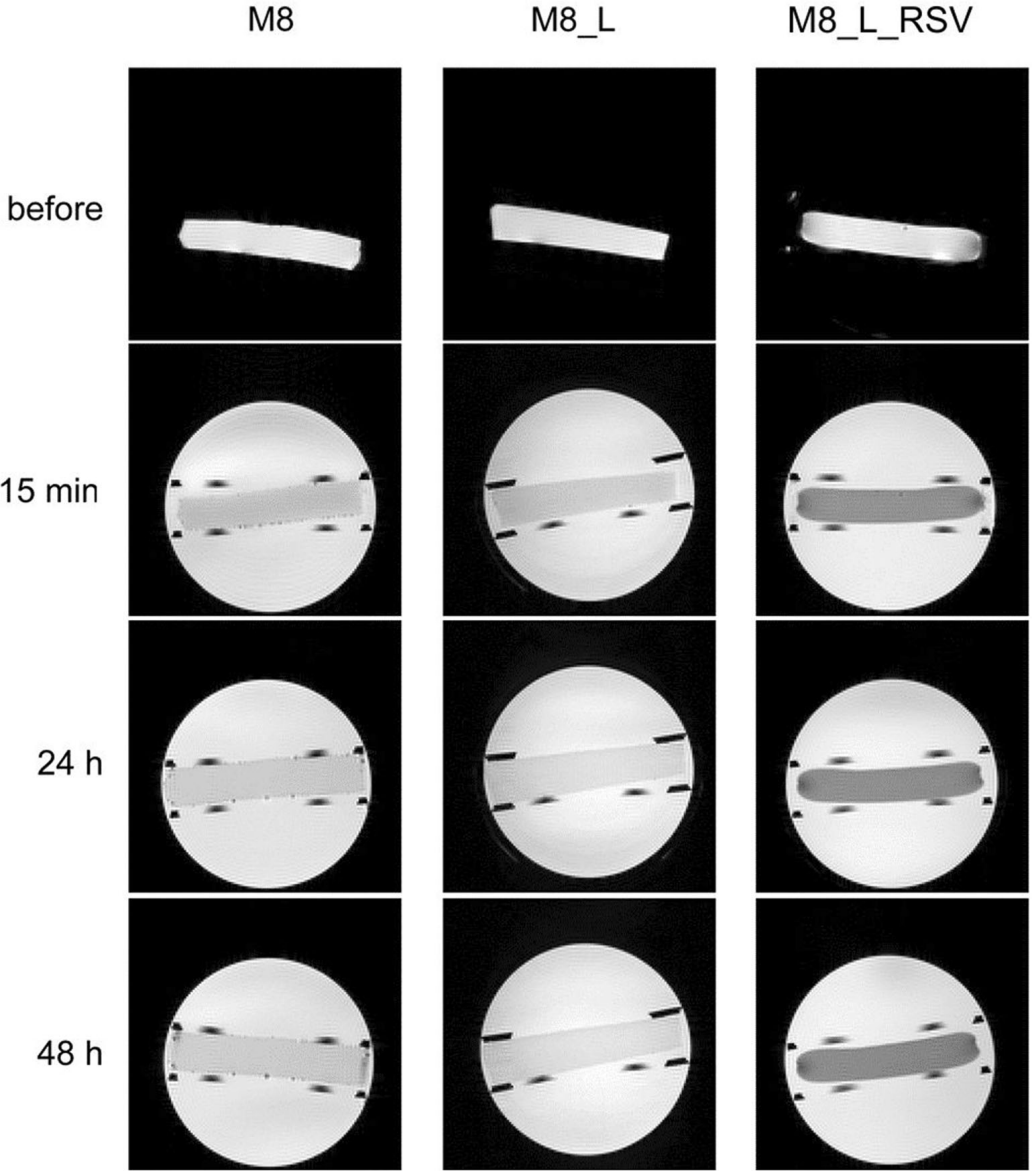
Selected MR images of cryogel membranes at various hydration times (TE = 10.5 ms)
